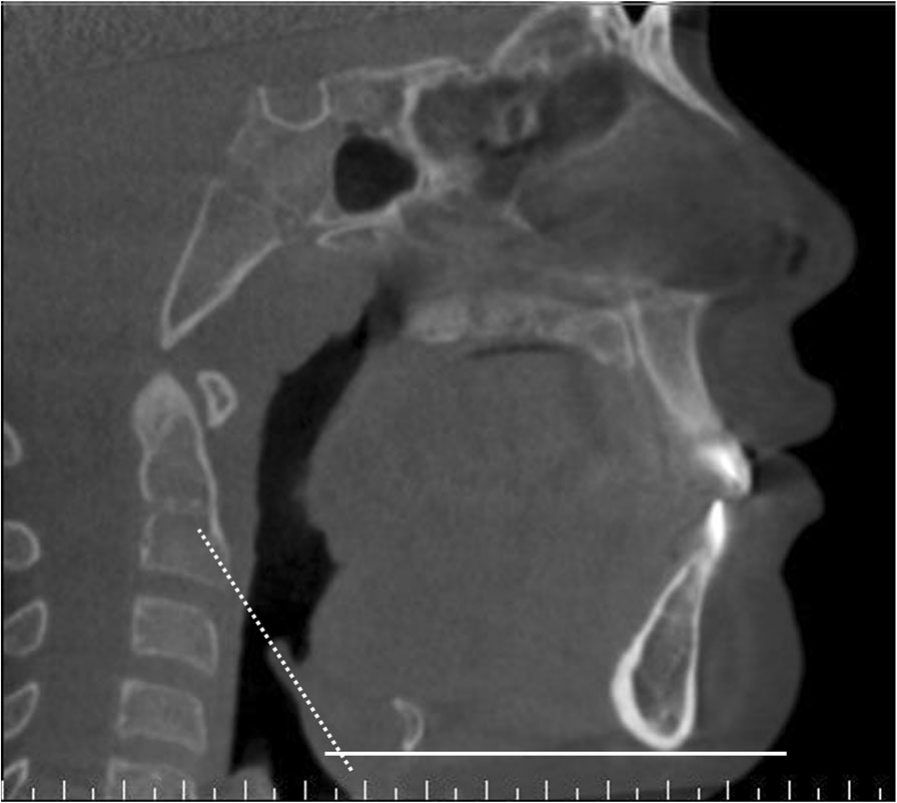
Sagittal CBCT image showing the inclination of epiglottis; the angle formed by the long axis of epiglottis (dotted line) relative to the horizontal plane (solid line)
